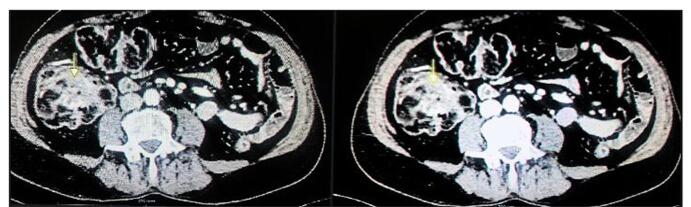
A computed tomographic (CT) scan of the abdomen with contrast showing telescoping of ileum into the cecum suggestive of ileocecal intussusception as indicated by the yellow arrows.
